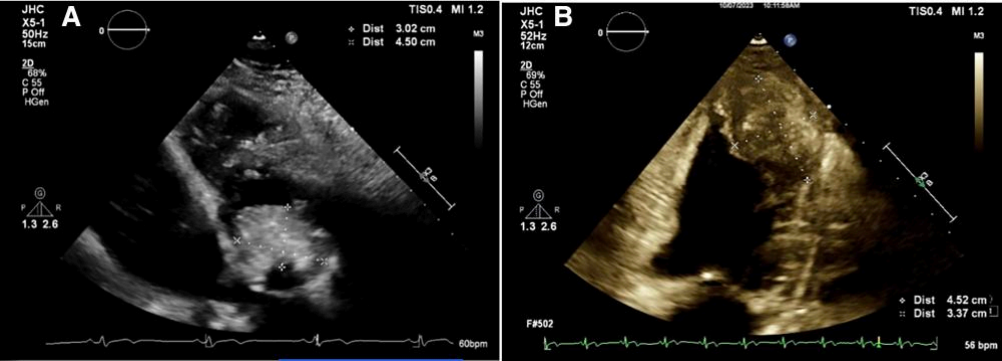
Transthoracic echocardiogram images. A flipped apical four-chamber view (*A*) demonstrating a 3.02 cm by 4.5 cm well-circumscribed mass within the apical pericardium. (*B*) An apical two-chamber view with a mass of 4.52 cm × 3.37 cm.
